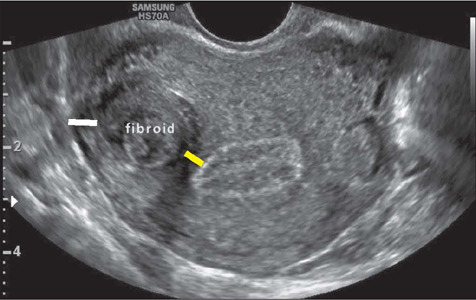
Transvaginal ultrasound image, in a cross-sectional view, showing an intramural
